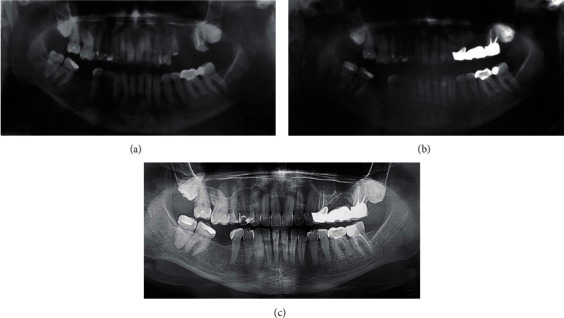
Panoramic radiograph: (a) preoperative radiograph showing bony defect at the postextraction site of tooth 2.6 and failing tooth 2.5; (b) radiograph taken 3 months after surgery showing bone repair; (c) radiograph taken at 8-year follow-up showing the stability of bone volume and absence of any pathological lesions.
